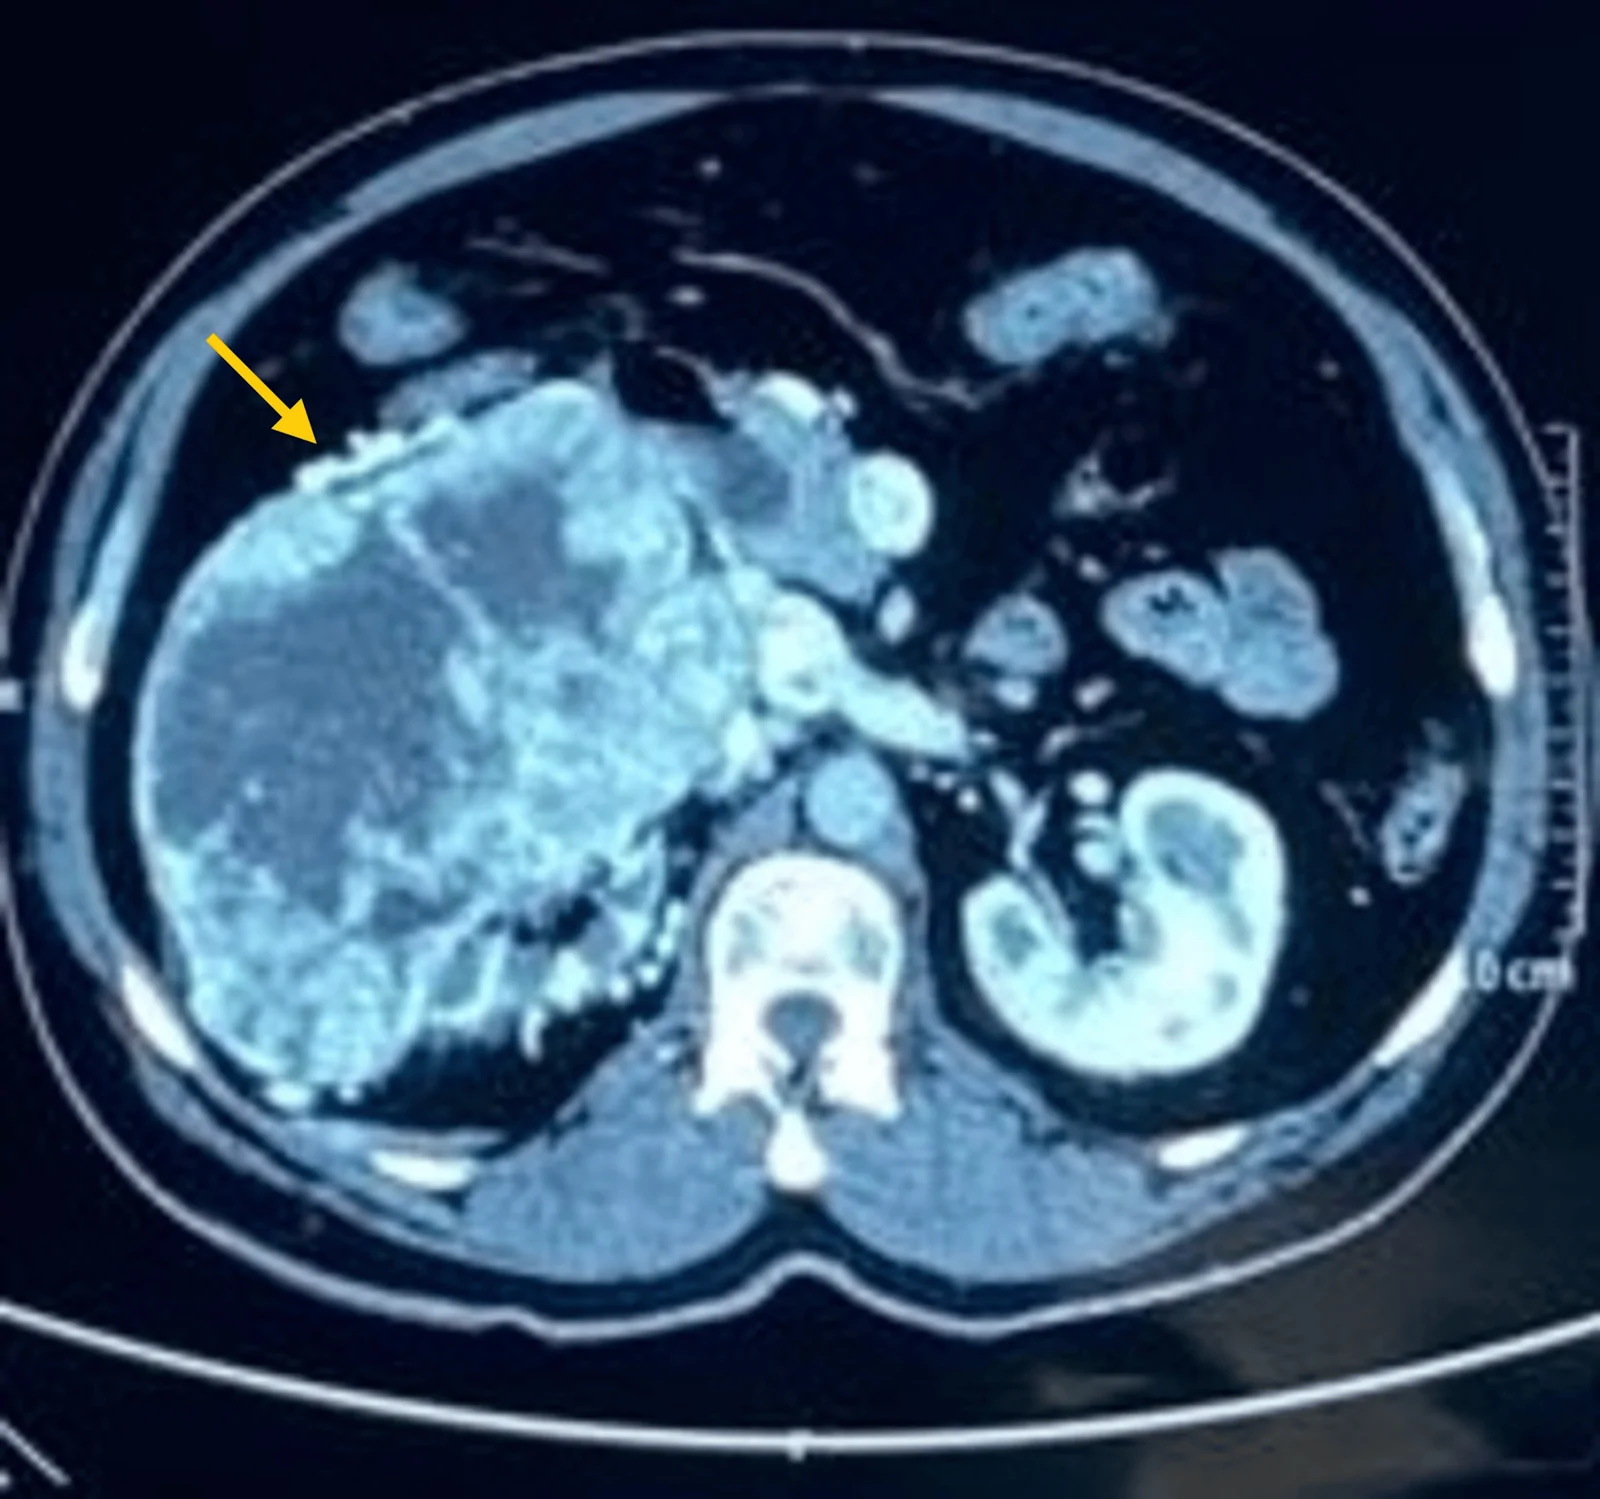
Preoperative axial contrast-enhanced computed tomography (CECT) of the abdomen and pelvis. The yellow arrow demonstrates a large right renal mass.
